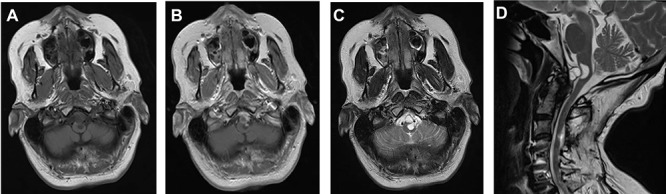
MRI findings of a ventral atlantoaxial degenerative cyst with an associated medullary syrinx; axial T1-weighted pre-contrast (A), axial T1-weighted post-contrast (B), axial T2-weighted (C) and sagittal T2-weighted (D) images demonstrate an approximately 1 × 1 cm T1-isointense, T2-hyperintense, rim-enhancing cyst at C1-2 compressing the ventral medulla with an associated a fluid-filled medullary syrinx.
